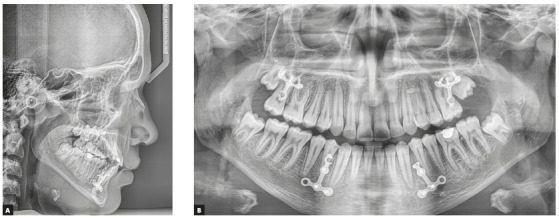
A) Final post-treatment lateral cephalometric radiograph. B) Final post-treatment panoramic radiograph.
